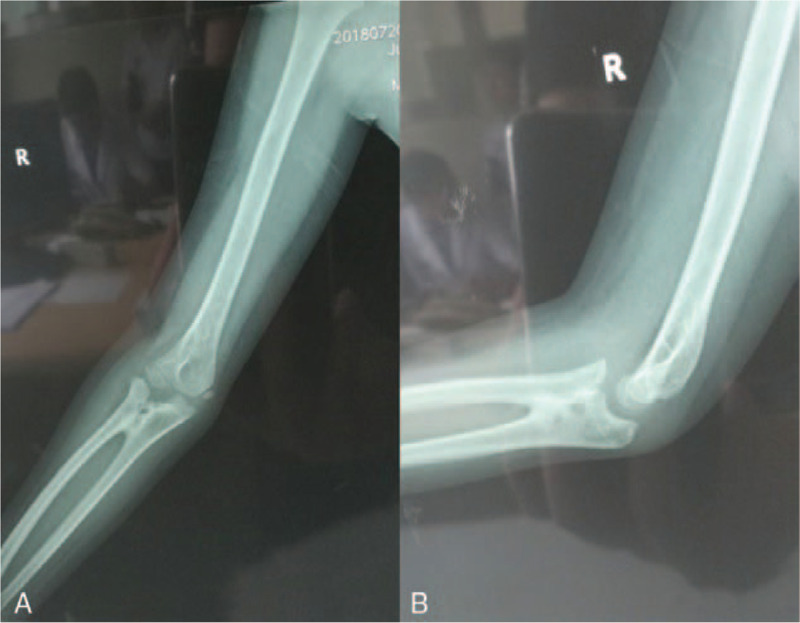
(A) X-ray of the anteroposterior elbow at the 6-mo postoperative follow-up. (B) X-ray of the lateral elbow at the 6-mo postoperative follow-up.
